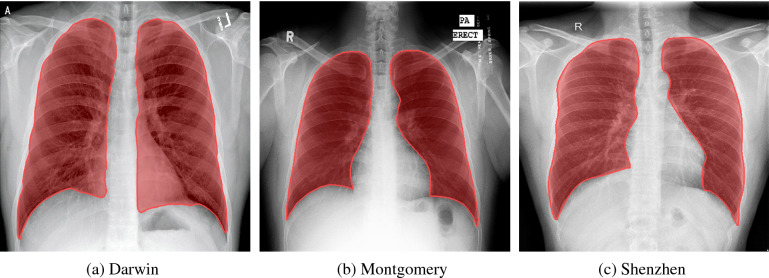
Examples of the collected chest X-ray images used for lung segmentation.
